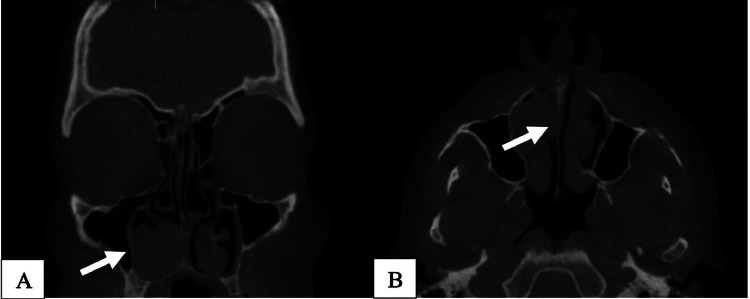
(A-B): CT findings of the facial sinus (Fig. 1A; left; coronal view) Expansive growing soft tissue mass of the inferior right turbinate measuring 2, 5 cm. No invasion of the skull or orbit is seen. (Fig. 1B; right; axial view) No signs of infiltration into other sinusoidal structures or bony erosion. Slight deviation of the nasal septum.
